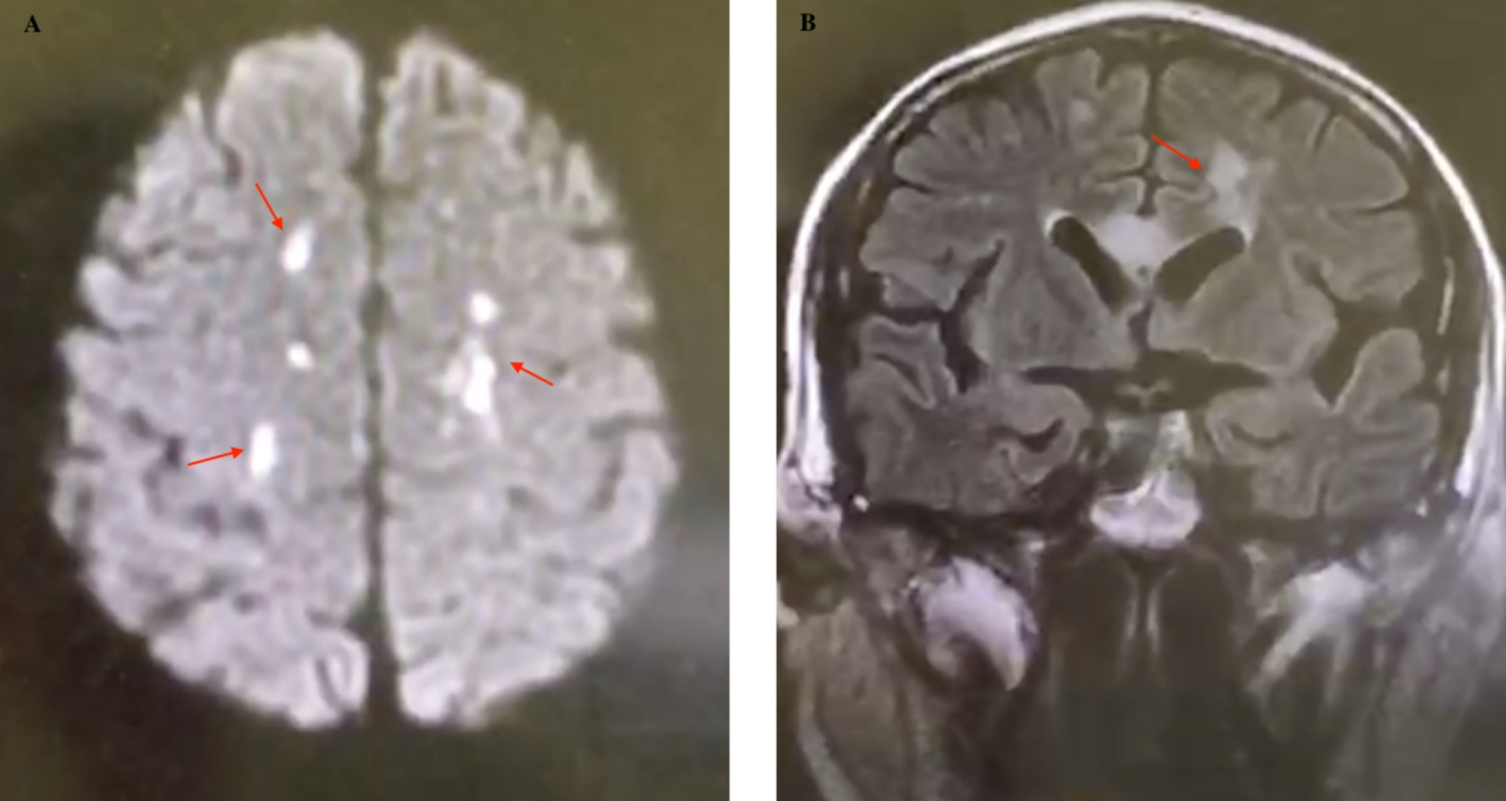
Brain MRI of patient 5 showed multiple focal lesions. A: multiple abnormal high signals in bilateral frontal lobes, semioval centre, and corpus callosum on DWI. B: multiple patchy and other slightly longer T1 and T2 signals in bilateral frontal lobes, semioval centres, and corpus callosum in the coronal image with no abnormal enhancement DWI, diffusion weighted image
